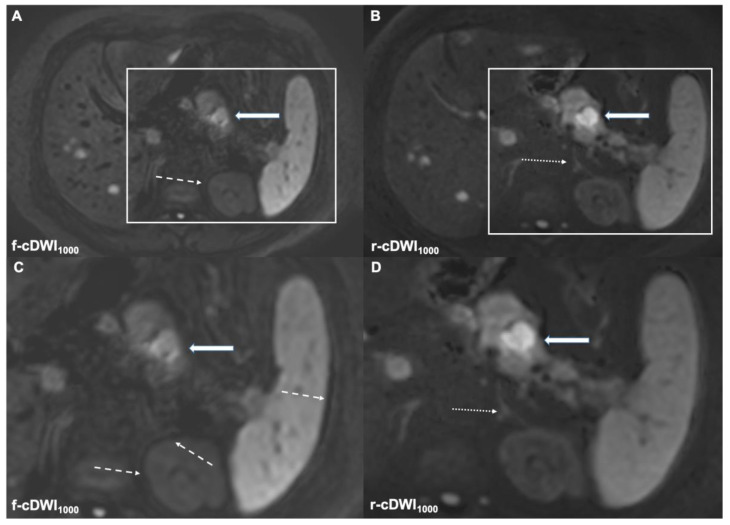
The high-resolution rFOV-DWI (B,D) yields better overall image quality compared to the conventional fFOV-DWI (A,C). Reduced distortion and motion artifacts enable better tumor delineation in the r-cDWI1000 (bold arrow in (B,D). Increased misregistration artifacts are depicted in the f-cDWI1000 images (dotted arrows in (A,C)). Additionally, note the better delineation of the left adrenal gland in the r-cDWI1000 images (dotted arrow in (B,D)).
